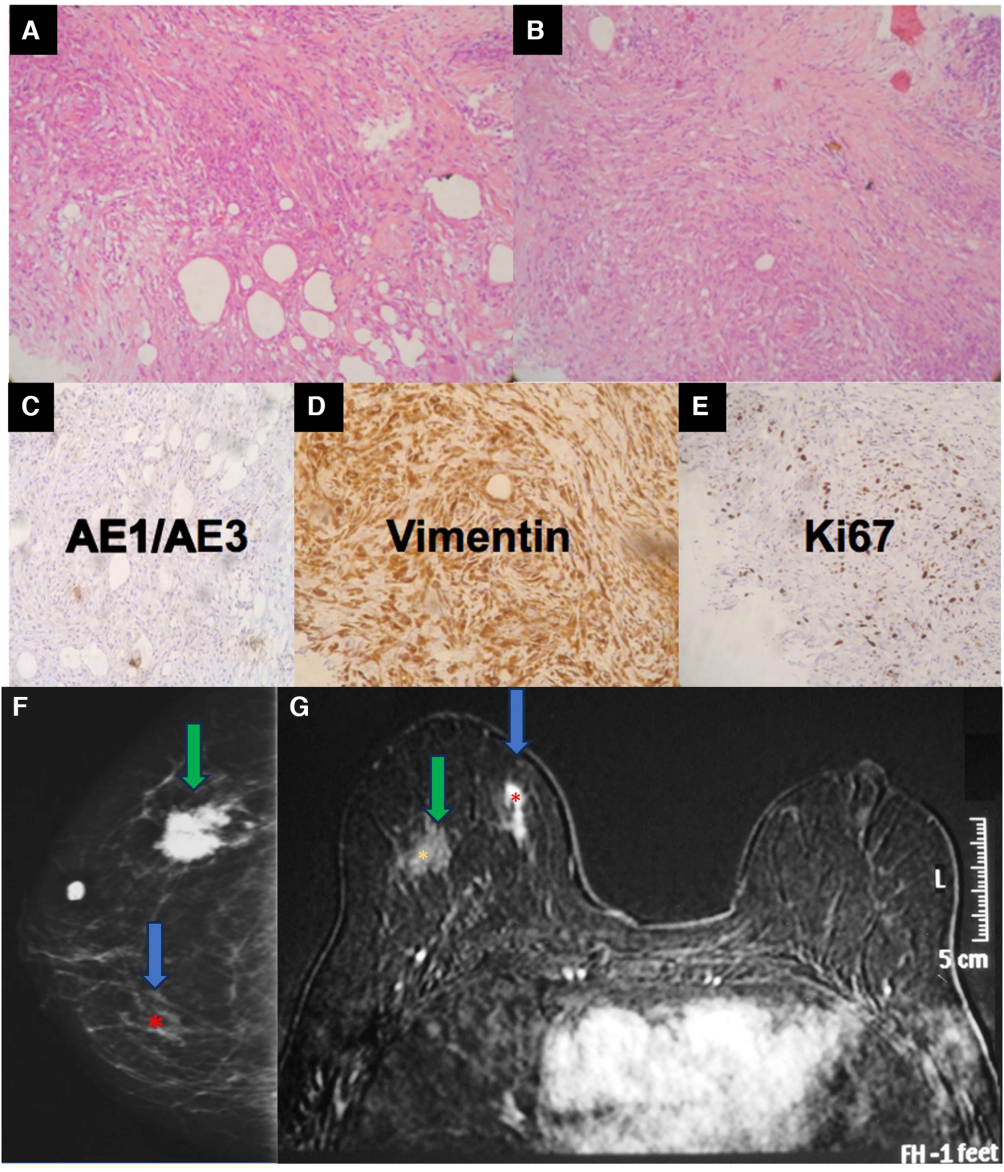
A 34-year-old woman with a history of industrial silicone injection for bilateral breast augmentation. (A,B) show the tumor composed of the spindle to epithelioid cells with a fibrohistiocytic appearance. The center is composed of more spindle cells. The tumor shows a negative reaction to AE1/AE3 (C), a strong reaction to vimentin (D), and an intermediate expression of KI-67 (E). Vimentin confirms the mesenchymal origin of the tumor. The mammography (F) shows a siliconoma (green arrow) as a spiculated mass and an architectural distortion in the site of the tumor (blue arrow). The MRI shows the early enhancement of the spindle cell tumor (blue arrow) (G).
